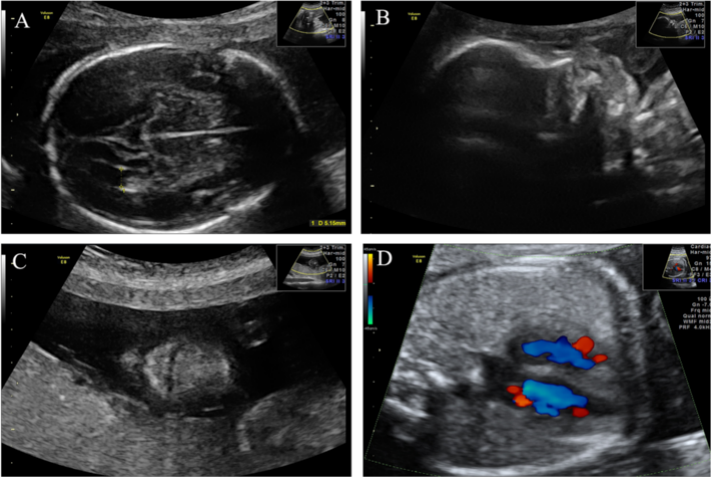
Detailed screening by ultrasound. No facial cleft or other structural abnormalities were observed in this fetus. Detailed ultrasound screening at 25 weeks of gestation show (a) a normal lateral ventricle view; (b) nasal bone view; (c) palate and lip view; and (d) apical four chamber heart view
